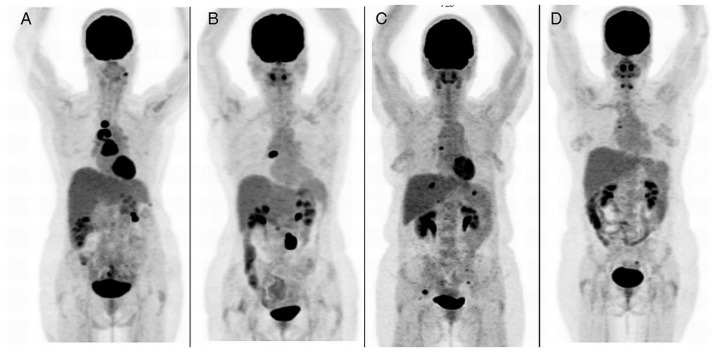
Patients with CA-125 levels within normal range with positive PET/CT scan showing supra-diaphragmatic lymph node and pelvic/abdominal metastases. CA-125 levels were (A) 13.8 U/ml; (B) 17.4 U/ml; (C) 20.8 U/ml; (D) 32.3 U/ml. PET, positron emission tomography; CT, computed tomography.
